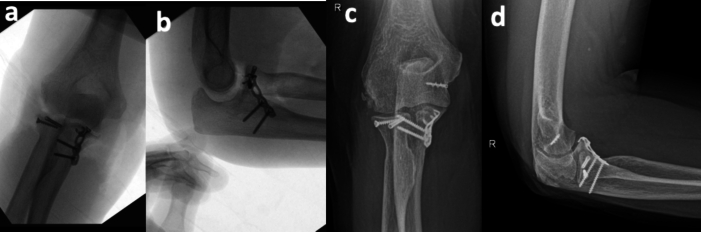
Patient case 1 Immediate intraoperative and postoperative images. a,b) AP and lateral fluoroscopic images showing adequate reduction but increased intra-articular space; c,d) AP and lateral radiographs with final result following repair of LCL and MCL.
